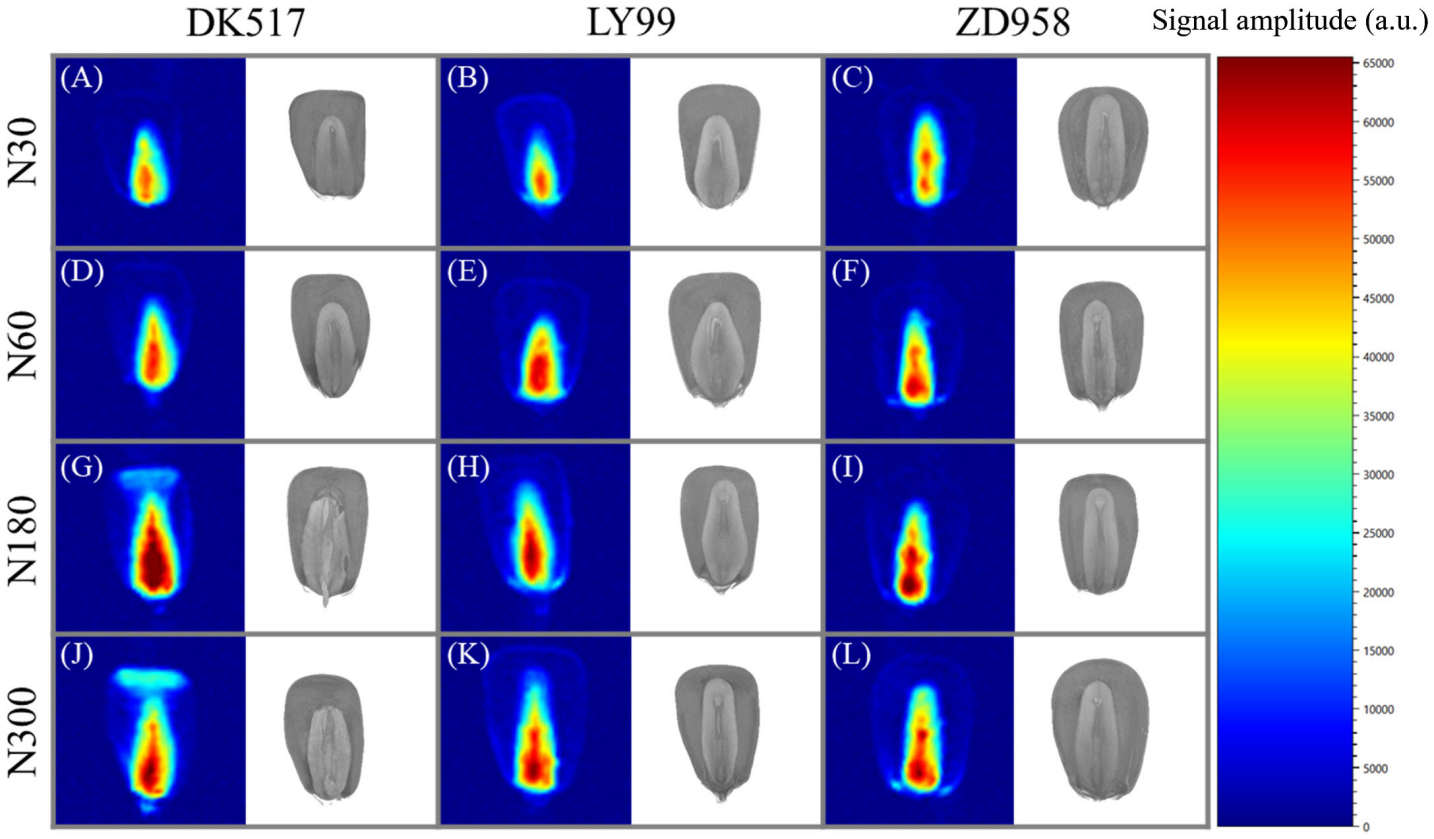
Visualization of moisture distribution and structural morphology in maize kernels after 18 hours of soaking based on LF-NMR and μ-CT scanning. (A–C) represent the moisture distribution at N30, (D–F) at N60, (G–I) at N180, and (J–L) at N300. The pseudocolor images represent the MRI scans of the kernels, while the grayscale images depict the μ-CT scans. The legend indicates the signal values corresponding to moisture content within the kernels.
